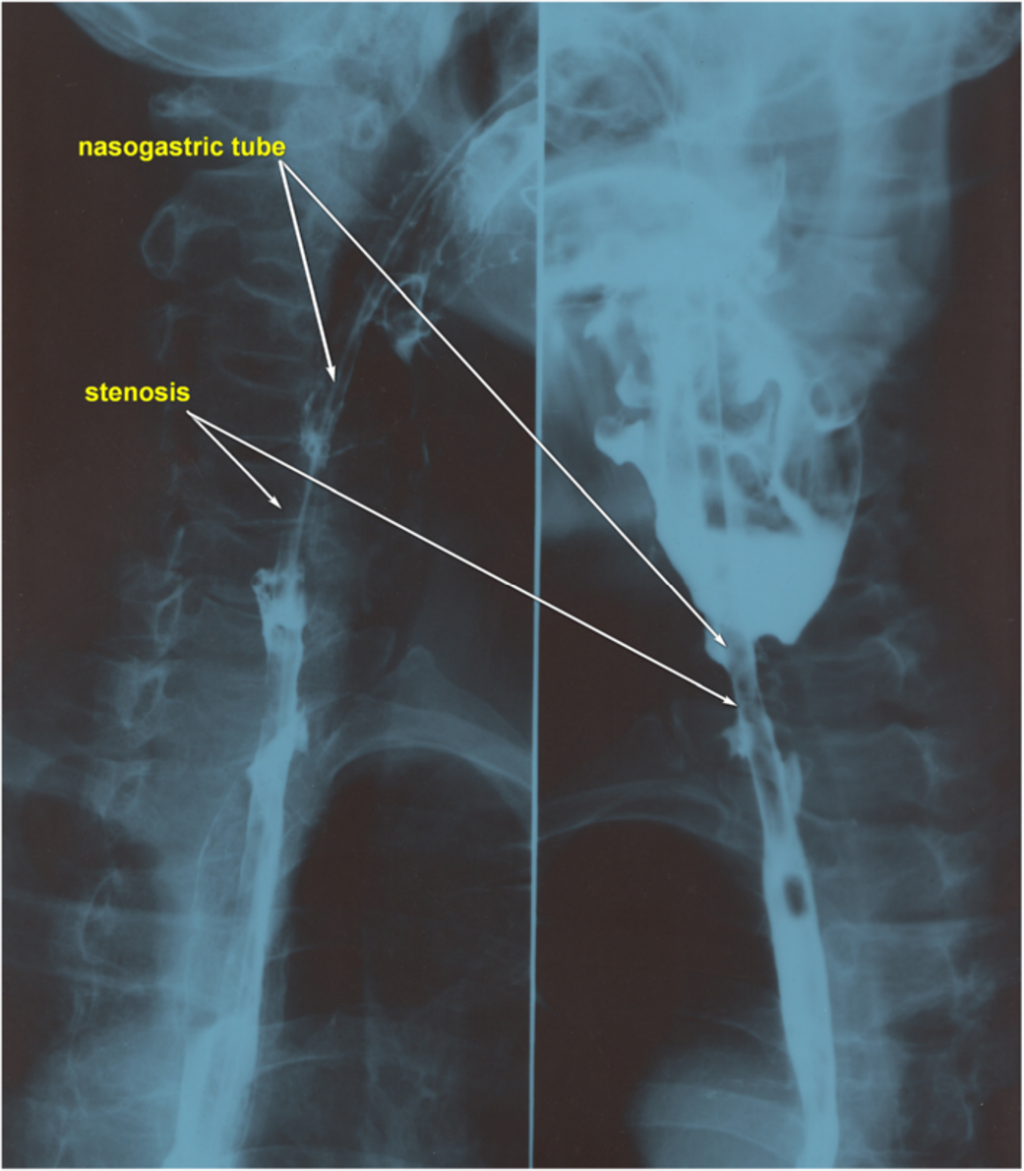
Esophageal barium radiography on the 7th postoperative day revealed severe stenosis of the cervical esophagus.
